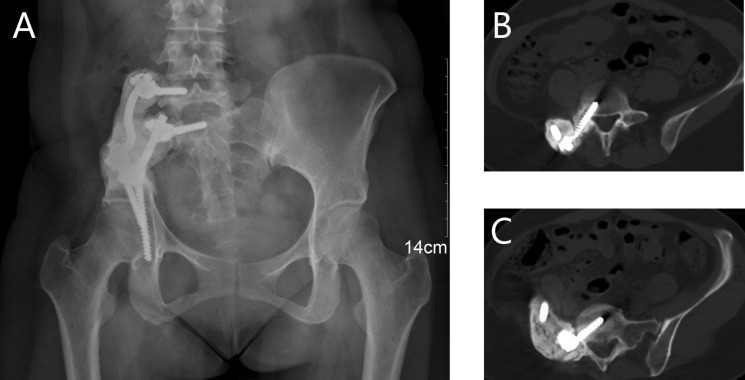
A 26-year-old female (case 1) with diagnosis of pelvis osteoclastoma affecting zone I (A) AP radiograph of the pelvis shows internal hemipelvectomy and subsequent pelvic ring reconstruction with screw-rod system and bone cement. (B, C) CT images show no evidence of tumor recurrence or implant loosening 144.1 months post-operatively.
